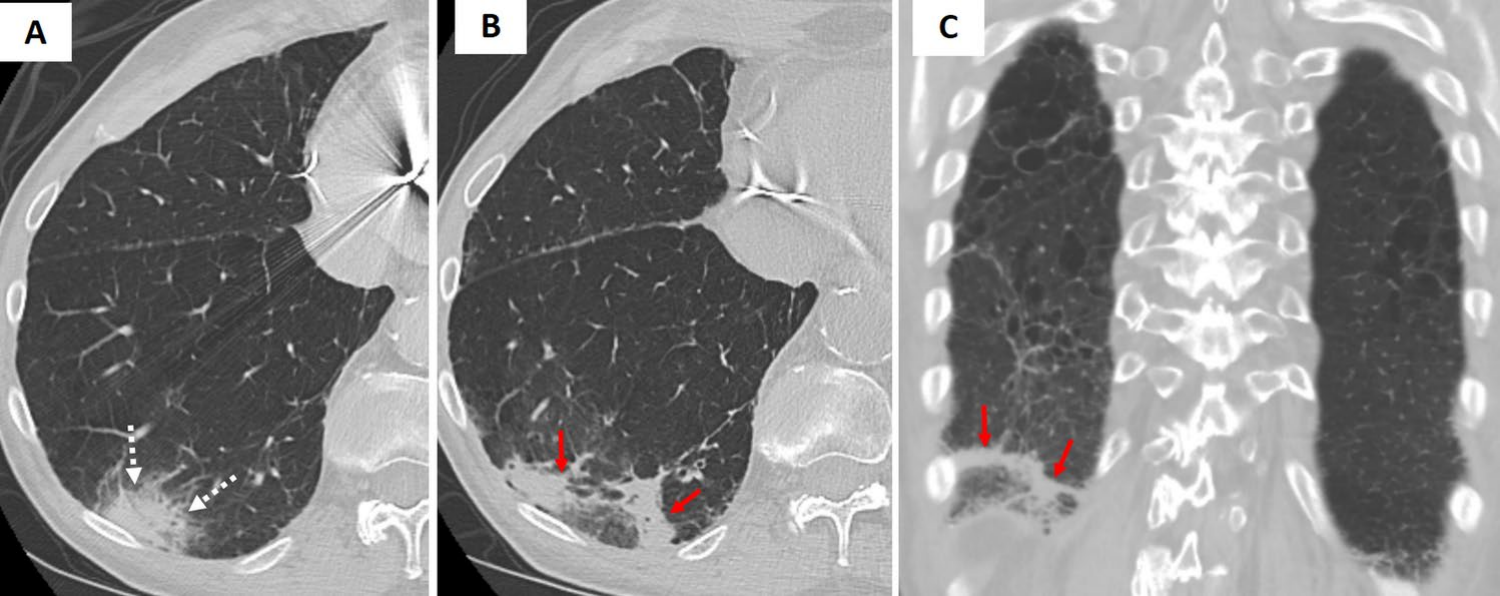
A Initial axial chest CT image demonstrates focal consolidation within the right lower lobe in a patient with COPD presenting with fever and cough. Sputum culture confirmed *Streptococcus pneumoniae* infection. B Axial and (C) coronal chest CT images obtained two weeks later reveal the development of the reverse halo (atoll) sign at the site of the prior consolidation
